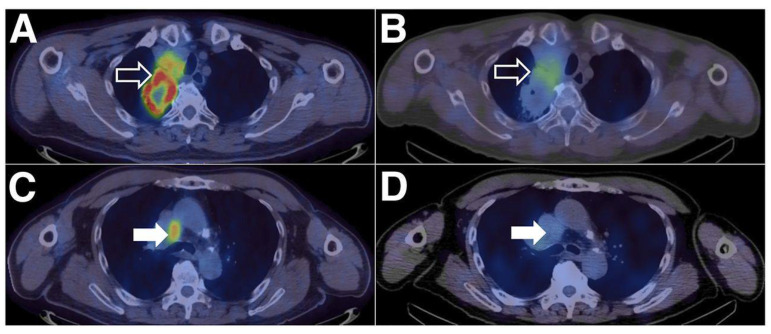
Heterogeneity in PD-L1 expression between primary and nodal sites of disease within the same patient. The right upper lobe tumor (open arrows) shows areas of high [^18^F]FDG uptake (SUVmax = 16.1) on PET/CT (A) and [^99m^Tc]Tc-NM-01 SPECT/CT (T:BP = 3.53) (B). The mediastinal lymph nodes (closed arrows) show high [^18^F]FDG uptake (SUVmax = 6.3) (C) but low [^99m^Tc]Tc-NM-01 activity (T:BP = 1.13) (D). The figure is reproduced with permission from [54].
